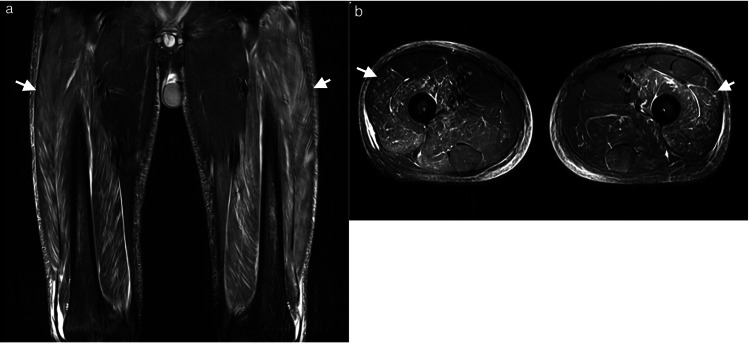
MRI findings on IST Day 40 (8 days after CsA discontinuation) (a) Short tau inversion recovery (STIR) coronal, (b) STIR axial Widespread STIR high signal areas in both thighs (white arrows), sparing the adductor muscles, with no bone abnormalities
